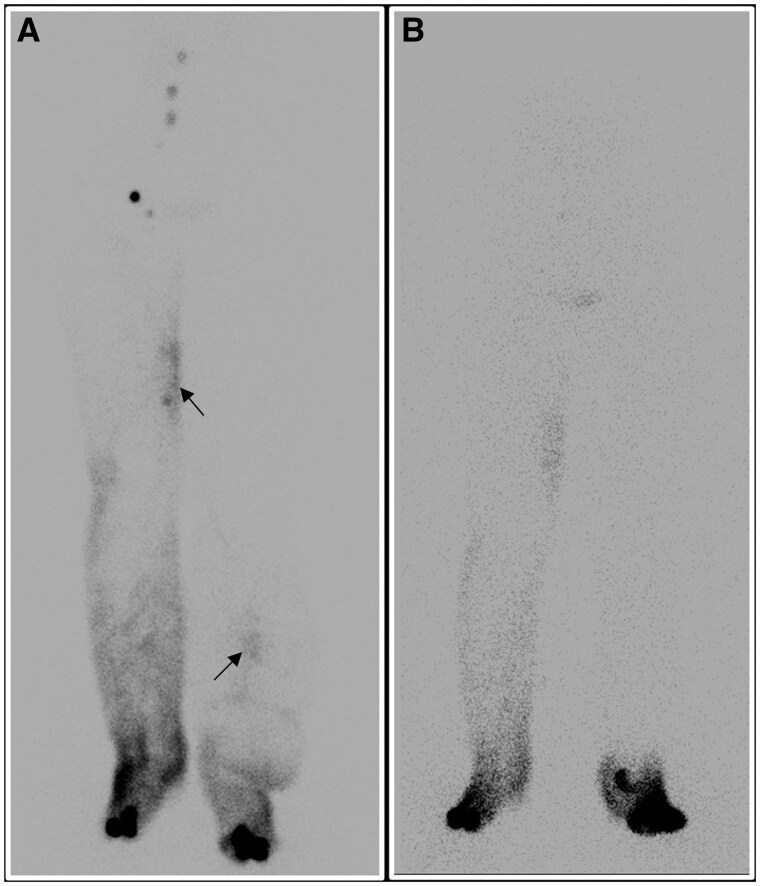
^99m^Tc nanocolloid lymphoscintigraphy done in a patient of carcinoma cervix with bilateral pedal oedema, (A) shows prompt visualization of right inguinal and pelvic lymph nodes, with significant dermal backflow in both the lower limbs (arrows). The patient underwent lymph node transfer surgery, however, 10 years post-surgery, the patient presented with a raw area over the dorsum of the foot. A repeat lymphoscintigraphy scan (B) was done which did not demonstrate the transferred lymph node in the left lower limb suggesting the non-viability of the lymph node.
